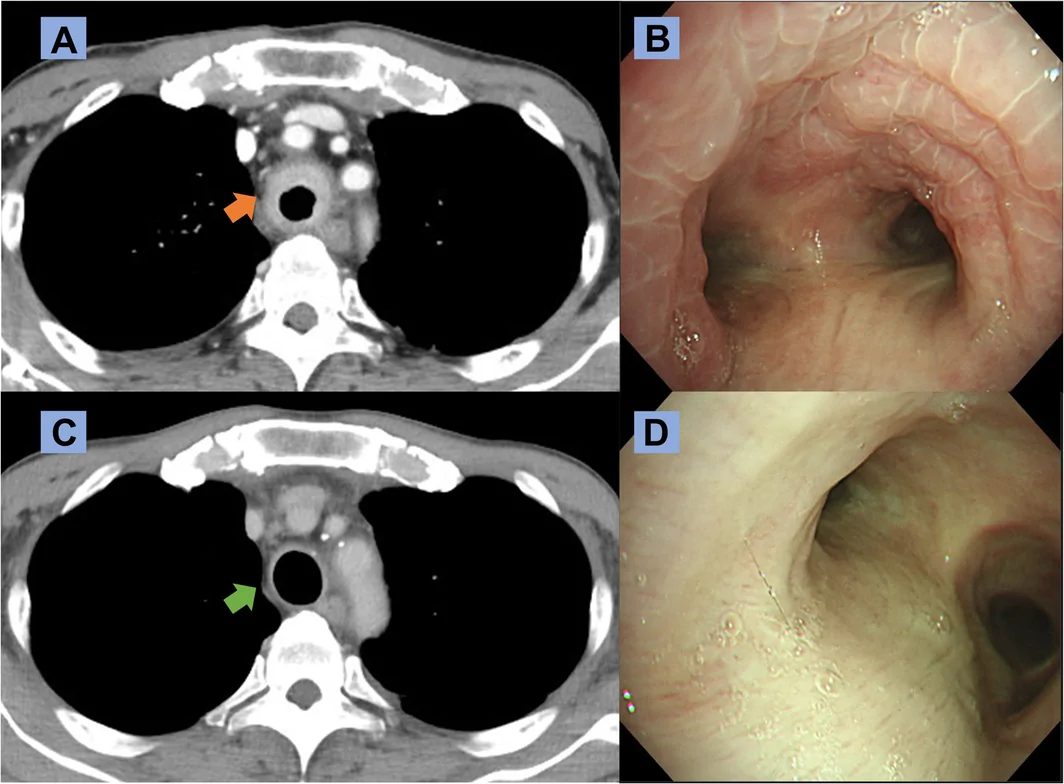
Pre‐treatment chest computed tomography and bronchoscopy show tracheal wall thickening and mucosal oedema (A, B, orange arrow). Post‐treatment imaging demonstrates improvement in tracheal wall thickening and mucosal findings (C, D, green arrow).
